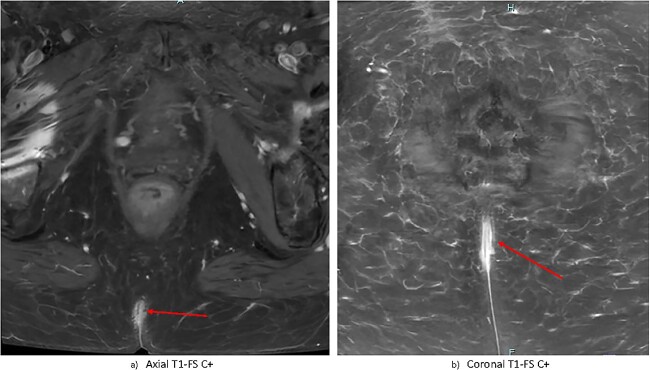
Gadolinium-contrast enhanced MRI images at time of diagnosis showing small-volume, superficial disease in (a) axial and (b) coronal planes. There is evidence of nonspecific superficial skin enhancement (arrows) but no evidence of significantly infiltrative disease or nodal metastases.
